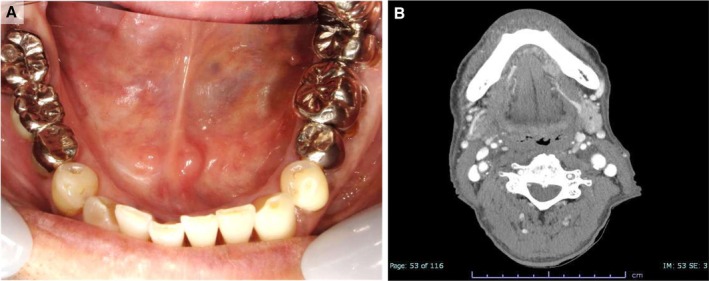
Post‐treatment intraoral and imaging findings. (A) Intraoral examination showed complete resolution of the tumor with no additional mucosal abnormalities. (B) Contrast‐enhanced coronal CT demonstrated no evidence of residual, recurrent, or metastatic disease.
